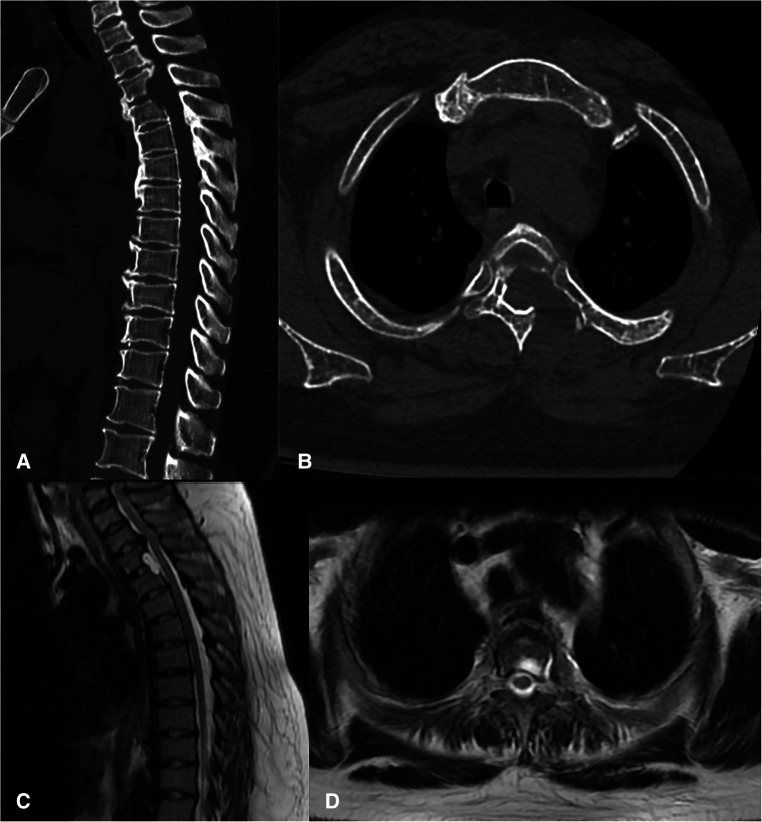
There was no evidence of instability or kyphosis on CT (A,B) and MRI (C,D) films 6 months after surgery and the thoracic spinal cord was completely decompressed.
